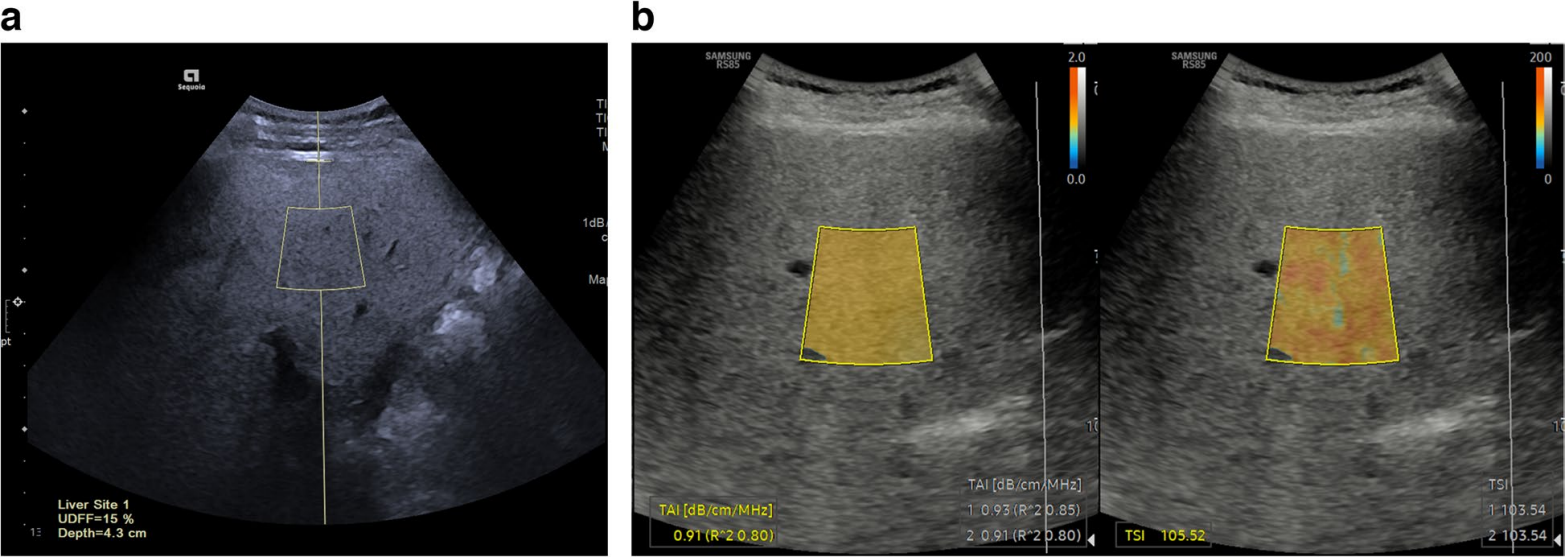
Measurements of ultrasound-based fat fraction measurements using different platforms. Ultrasound-based fat fraction values were measured in each patient using ultrasound-derived fat fraction (UDFF, Siemens Healthineers (a)) and quantitative ultrasound-derived estimated fat fraction (USFF, Samsung Medison (b))
